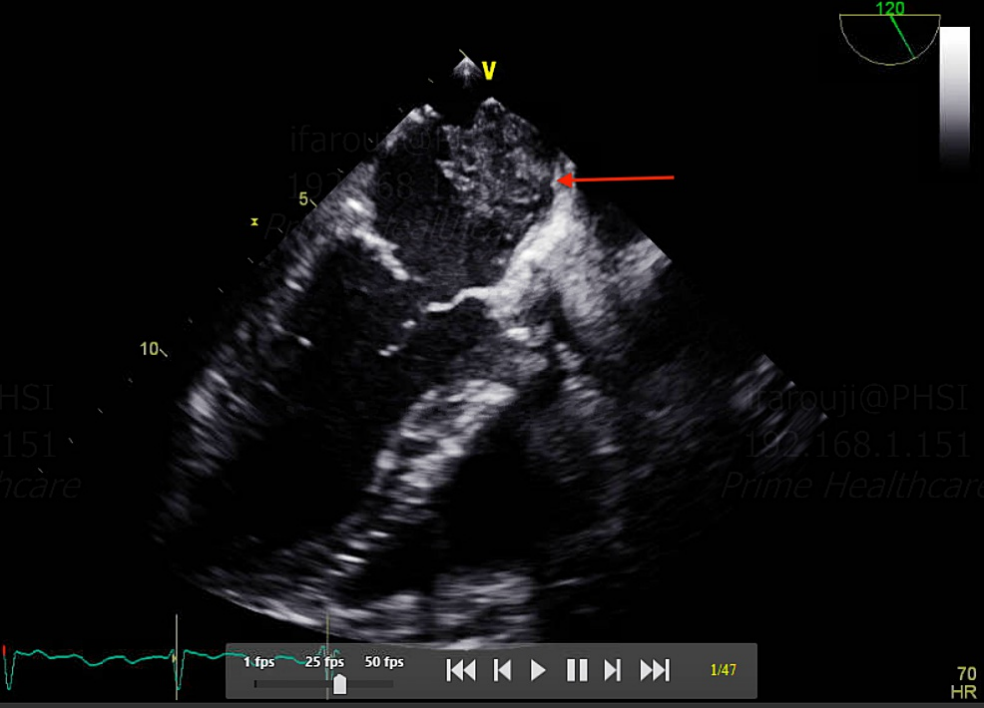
TEE showing a thrombus in the left atrium (red arrow) TEE: transesophageal echocardiogram
